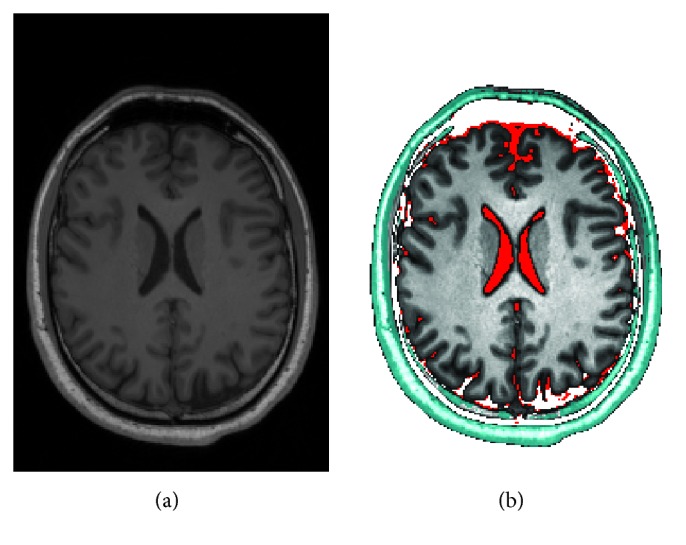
Slice of a brain MRI scan. (a) is normalized but otherwise unprocessed. (b) is segmented into skull/skin (teal), cerebrospinal fluid (red), and brain (grey) tissue. The brain tissue is contrast-enhanced.
